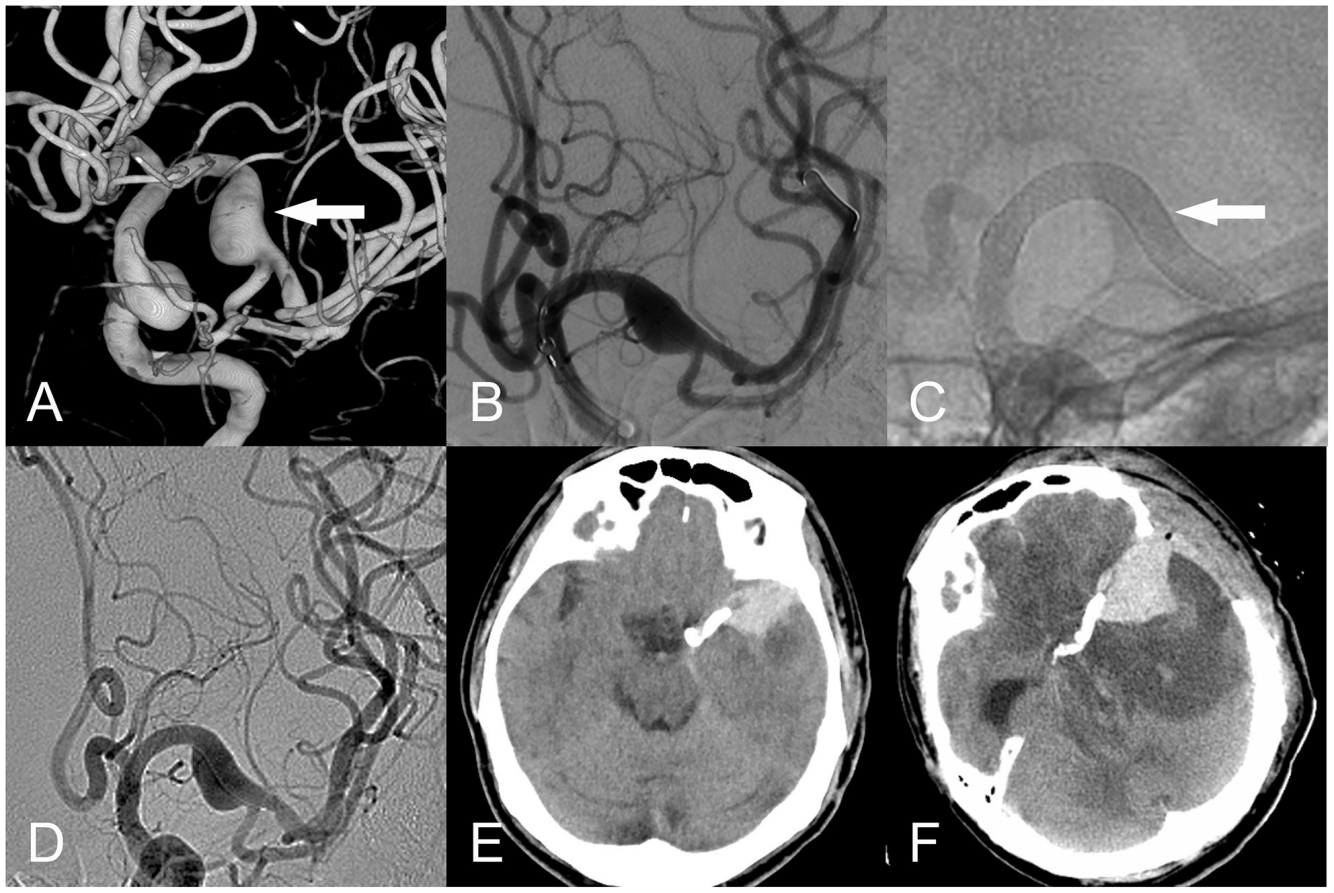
Fusiform aneurysm of the left middle cerebral artery. (A) Three-dimensional reconstruction (white arrow). (B) The PED was implanted intraoperatively uneventfully (white arrow). (C,D) The PED was well adherent, and the contrast was obtained after the release of the PED. (E) Nine hours postoperatively, the patient developed severe headache. CT suggested cerebral hemorrhage with subarachnoid hemorrhage, and decompressive bone flap was performed urgently. (F) 1 day after decompressive surgery by deparaffinization flap, CT suggested significant brain edema around the intracerebral hemorrhage.
